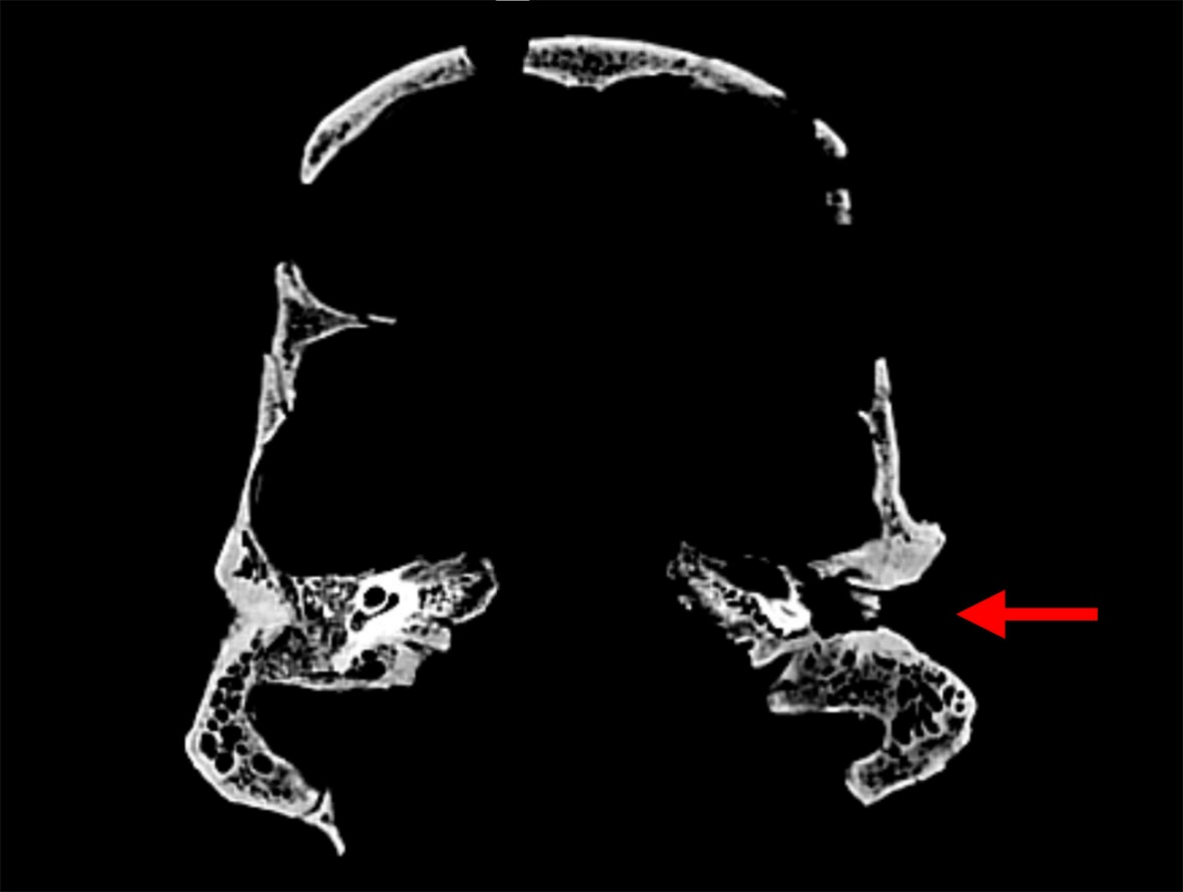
CT-scan aspect of left auricular canal exostosis of skeleton 19.2 with a partial closing of the external ear canal (red arrow / picture P. Charlier)
